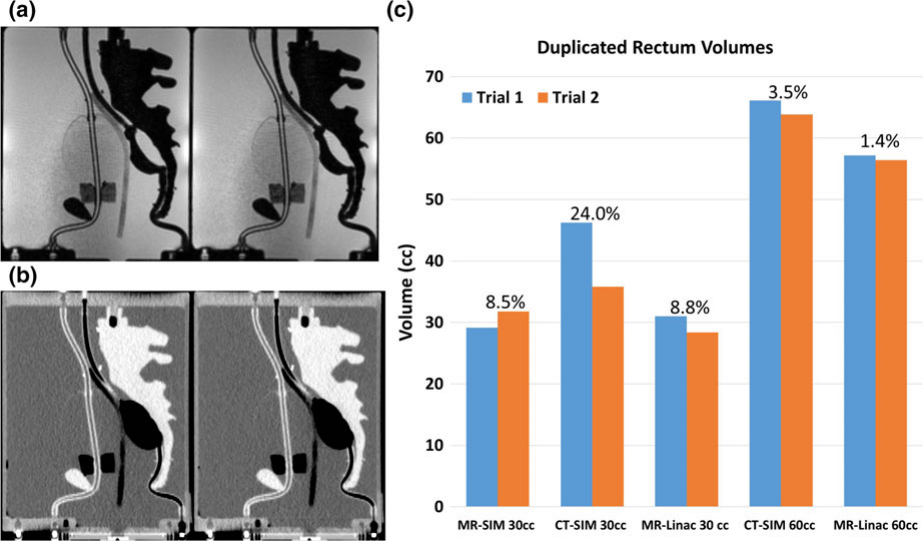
Reproduced sagittal volumes: (a) MR‐Linac 30 mL and (b) CT‐SIM 60 mL datasets for reproduced rectum trials. (c) Volume changes for the bladder and rectum from trials 1 and 2 of duplicated rectal volumes in each modality, with the corresponding percent volume changes.
